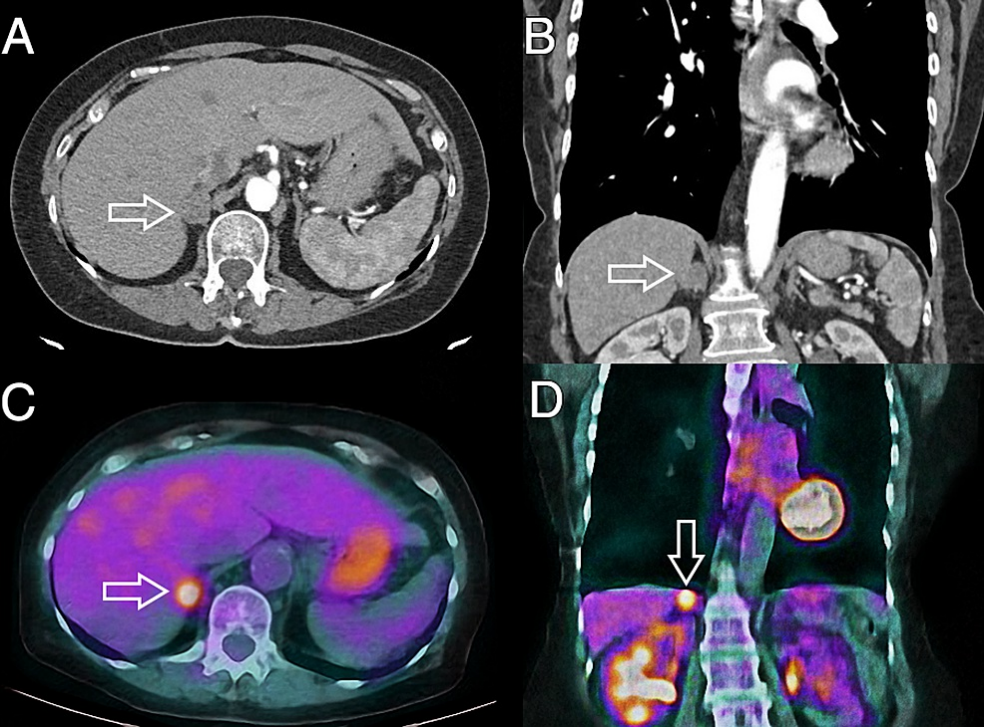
Follow-up diagnostic imaging A) axial and B) coronal computed tomography (CT) images with arrows pointing at the right adrenal tumor. Characteristics of the mass include heterogeneous textures and irregular borders raising radiological suspicion for possible malignancy C) axial and D) coronal positron emission tomography/computed tomography (PET/CT) images with arrows pointing at the right adrenal tumor
